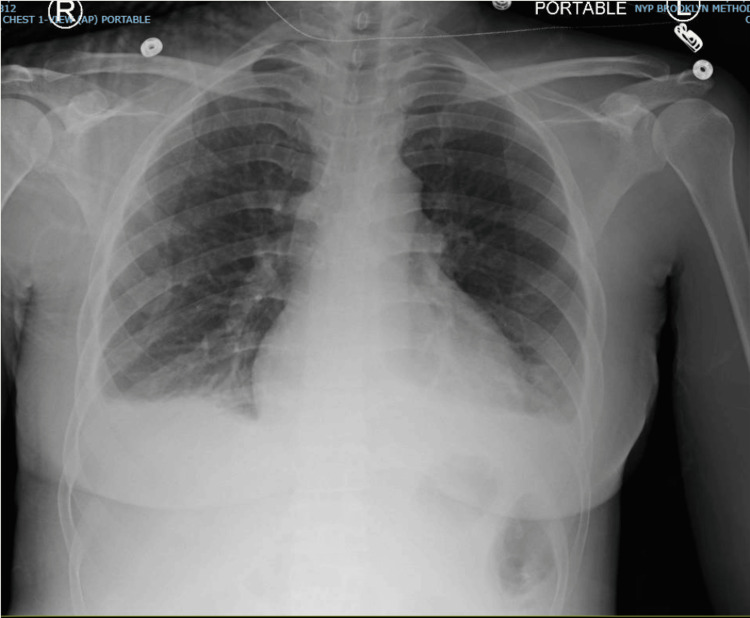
Chest X-ray of case 1 showing right lower lobe consolidation
